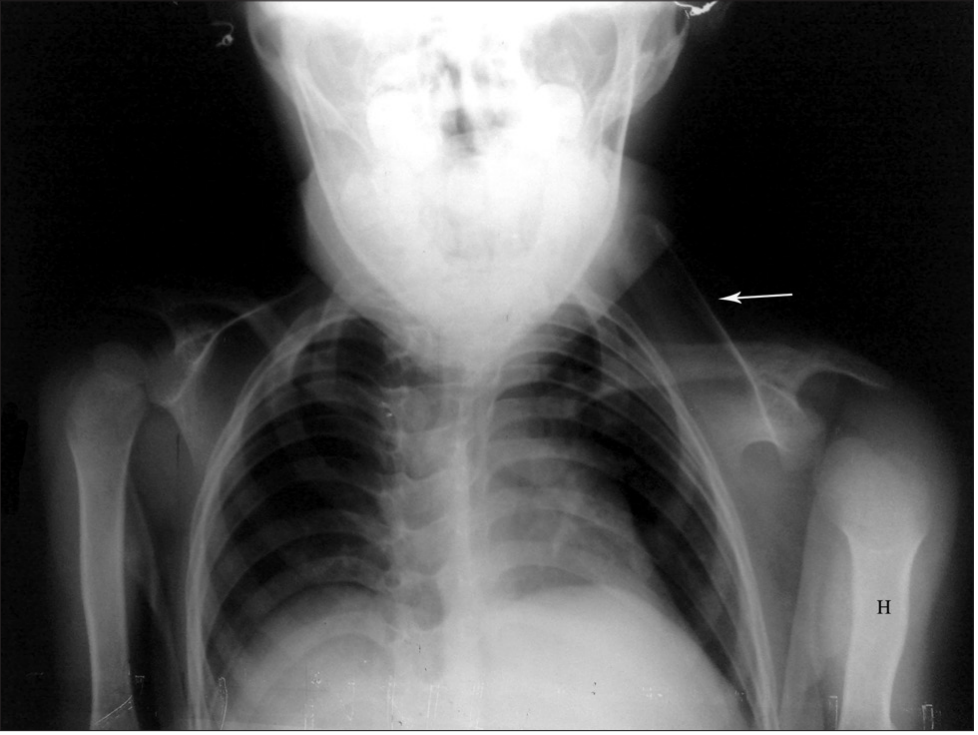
Oblique radiograph (arrow – omovertebral bar; H – Hypoplastic humerus)
